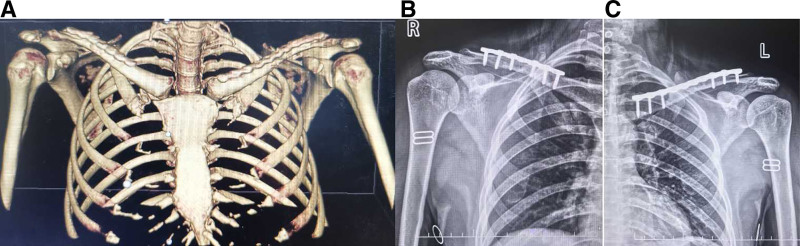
Postoperative 3-dimensional CT reconstruction of the chest after open reduction and internal fixation and X-rays of both shoulder joints 2 months after surgery. CT = computed tomography.
